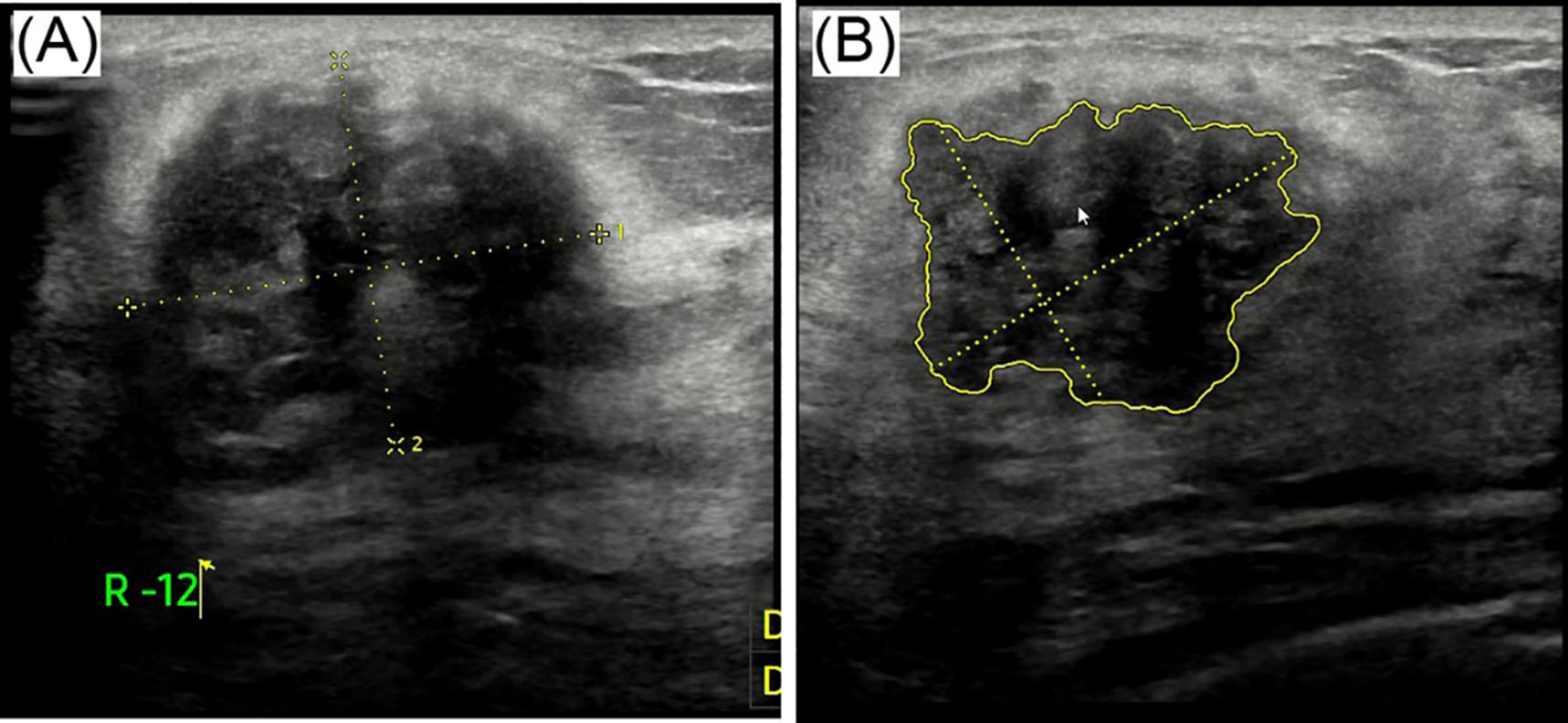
Ultrasound images labeled (A) and (B). Image (A) shows a mass with dotted yellow lines indicating measurements. Image (B) highlights an irregularly shaped area outlined in yellow, also marked with measurement lines.
